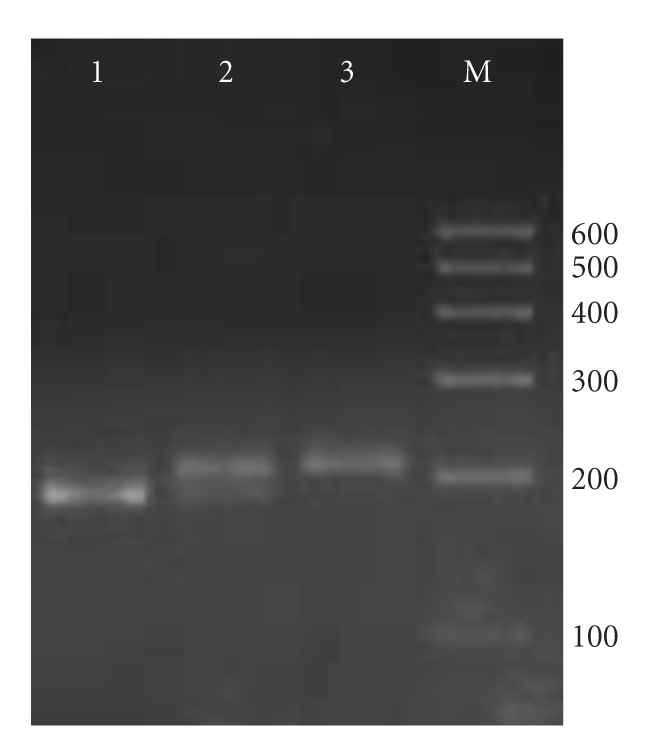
PCR-RFLP assay for analyzing the +781 C/T polymorphism of IL-8. Lanes 4 and 5 of heterozygous CT genotype showed three fragments of 203, 184, and 19 (not shown) bp; lane 1 and 3 of homozygous TT genotype showed only one fragment of 203 bp; lanes 2 and 6 of homozygous CC genotype showed two fragments of 184 and 19 (not shown) bp. Lane M was loaded with 100–600 bp DNA markers.
